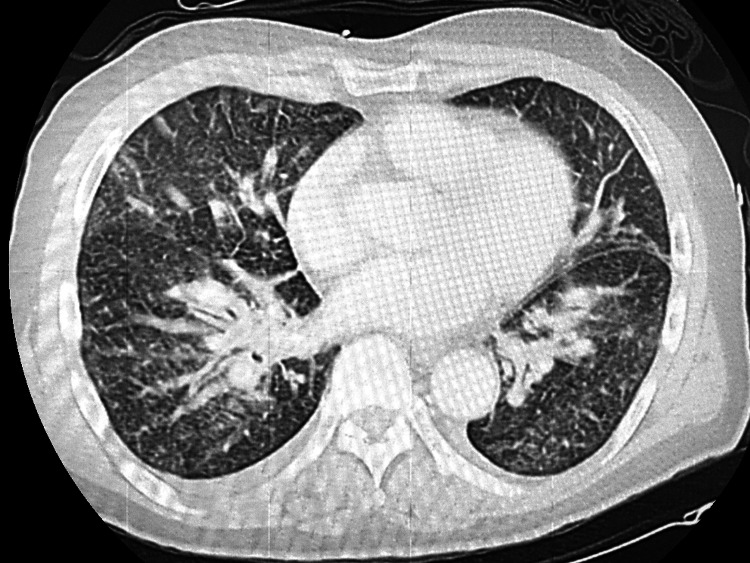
Computed tomography (CT) of the chest on admission. Contrast-enhanced axial image of the chest demonstrating increased pulmonary vascular markings and mild peribronchovascular thickening.
